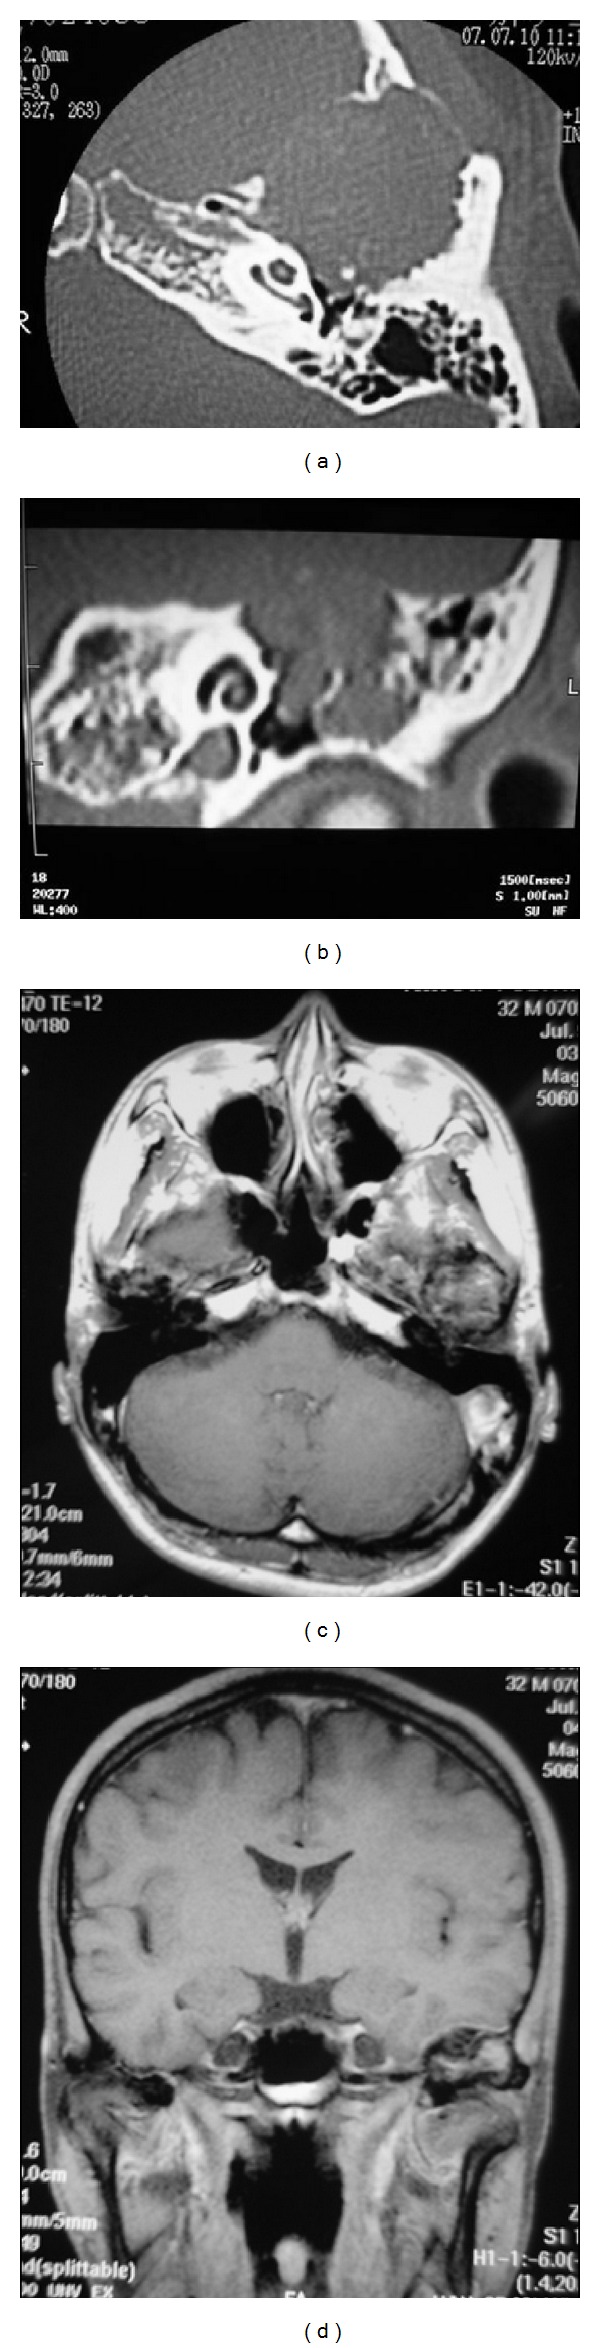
Temporal bone computed tomographic scan shows soft tissue shadow from the bone destruction with massive extension to the epitympanum and ossicular chain (a). In the coronal section, the tumor invades the skull base, and the outline of the tumor shows osteosclerosis with decalcification (b). Magnetic resonance imaging of the head revealed a mass 3 cm in diameter at the left temporal bone that was unequally enhanced after intravenous gadolinium (c). At the coronal section, the border with the tumor and the left mandible head was indistinct. In addition, the dura mater of the middle cranial fossa showed enhancement (d).
